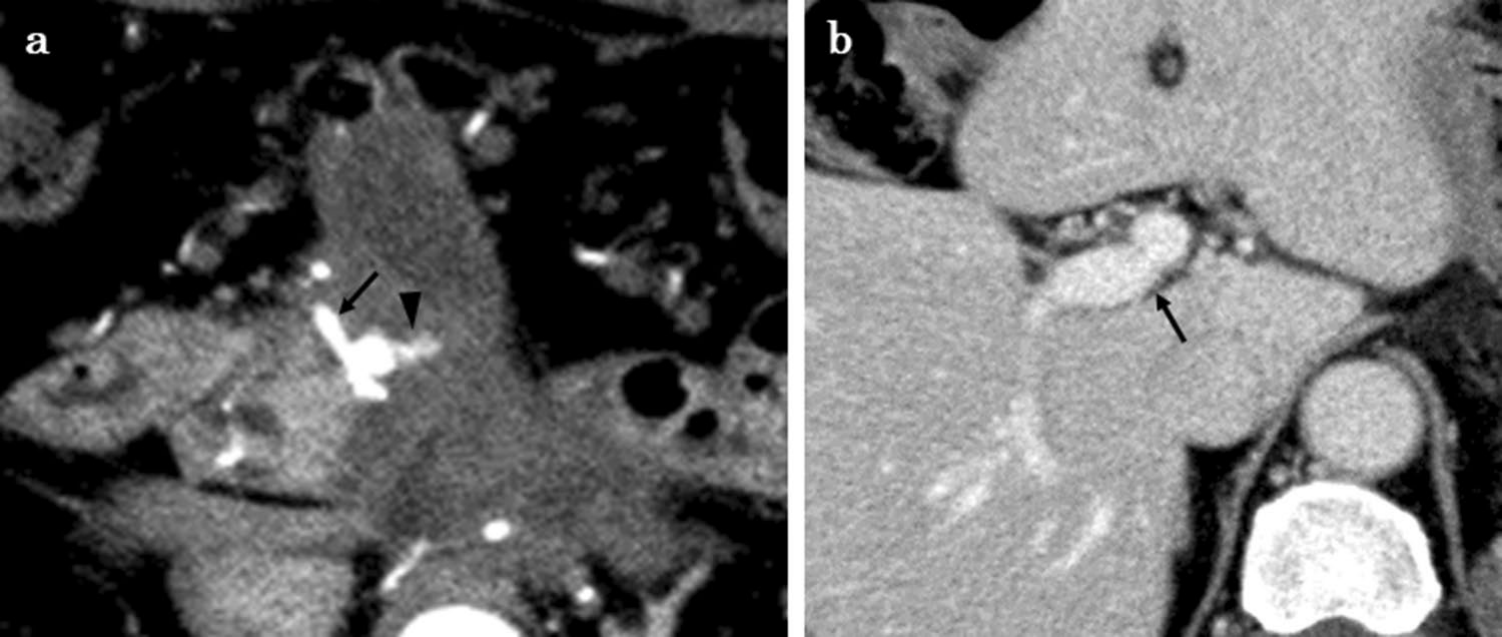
Enhanced CT findings six months after surgery. a: Enhanced CT showed a pseudoaneurysm arising from the ASPDA (black arrow) and asymptomatic active bleeding arising from the ASPDA pseudoaneurysm (black arrowhead). b: Enhanced CT showed that the blood flow of the PV was maintained (black arrow). *ASPDA* anterior superior pancreaticoduodenal artery, *CT* computed tomography, *PV* portal vein
